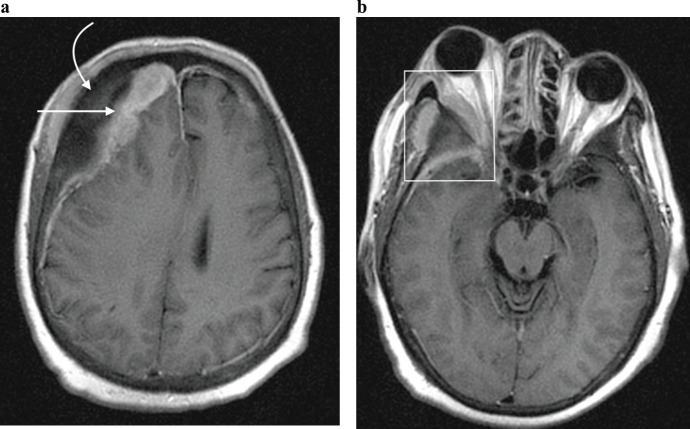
Axial post contrast T1-weighted MRI image in a 65-year-old man with known skeletal bone metastases from prostate cancer, demonstrating (a) enhancing right dural based metastases (straight arrow) underlying right frontal bone osseous metastases (curved arrow); and resultant effacement of the right lateral ventricle with evident midline shift to the left. (b) Same patient as in (a), at the level of the orbits. A right orbital metastatic lesion is demonstrated (rectangle) centred on the greater wing of sphenoid bone, with a contiguous soft tissue mass extending into the temporal fossa as well as the lateral extraconal space, with associated proptosis.
